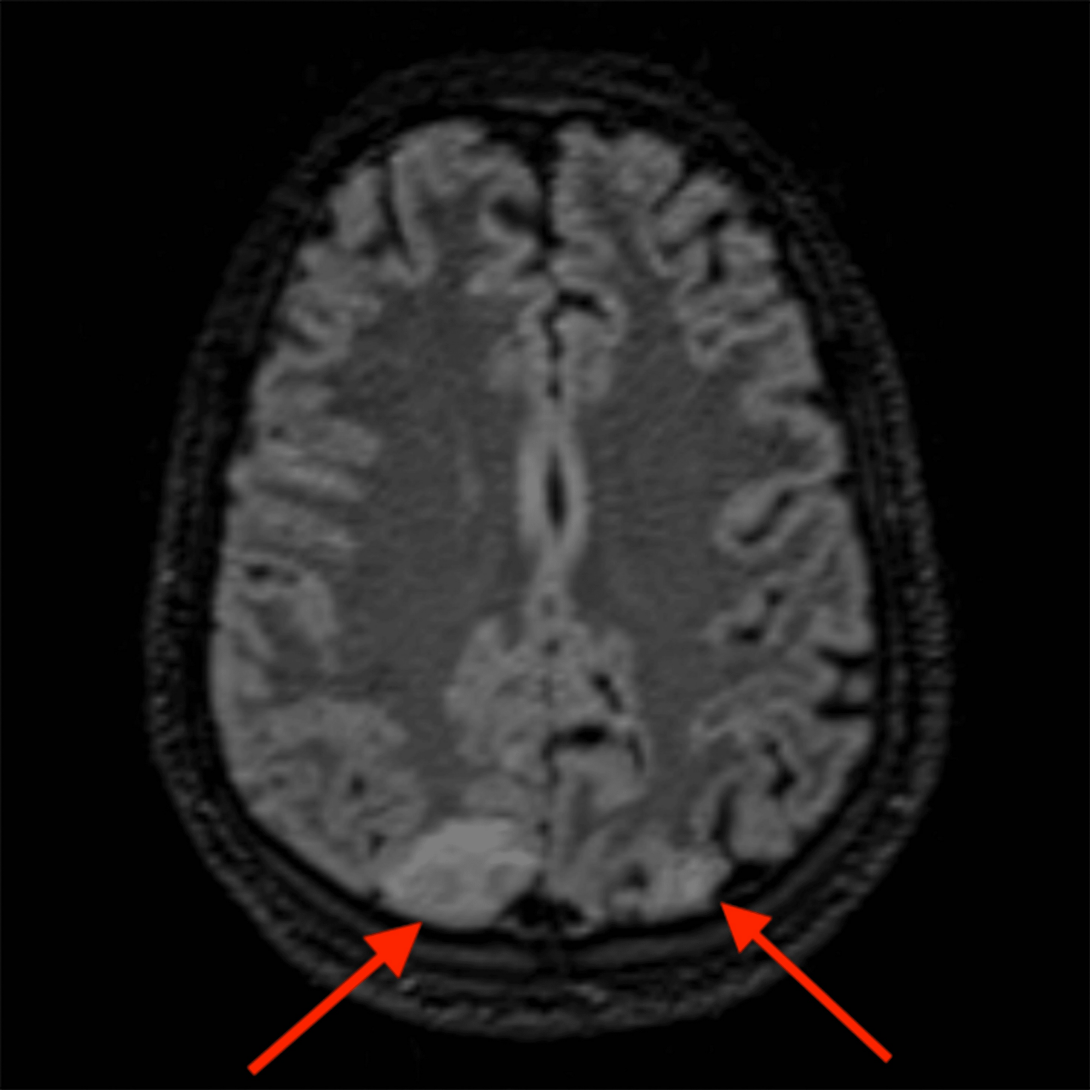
Axial FLAIR image of Patient 2 (MN) showing hyperintensities (arrows) in bilateral posterior parietal regions The red arrows point to bilateral posterior parietal regions with patchy FLAIR hyperintensities, consistent with PRES FLAIR: fluid-attenuated inversion recovery; PRES: posterior reversible encephalopathy syndrome
